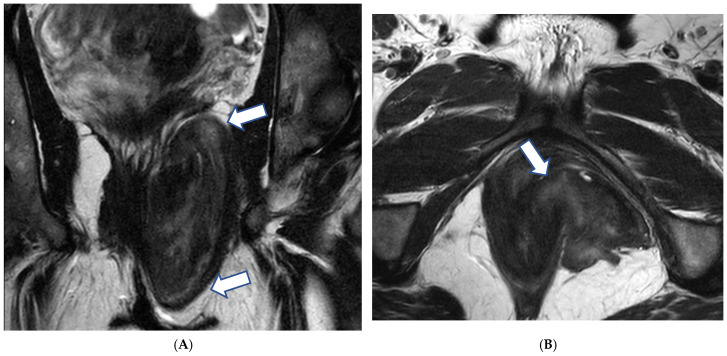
A 39-year-old woman, six months after a c-section presented with a left palpable vulvar mass. T2-weighted images in the coronal (A) and axial (B) plane demonstrate a large low T2 signal intensity mass occupying the left ischiorectal fossa (arrows in (A)) also invading the left side of the external sphincter (arrow in (B)). Biopsy showed the presence of a tumor of mesenchymal origin consistent with aggressive angiomyxoma.
